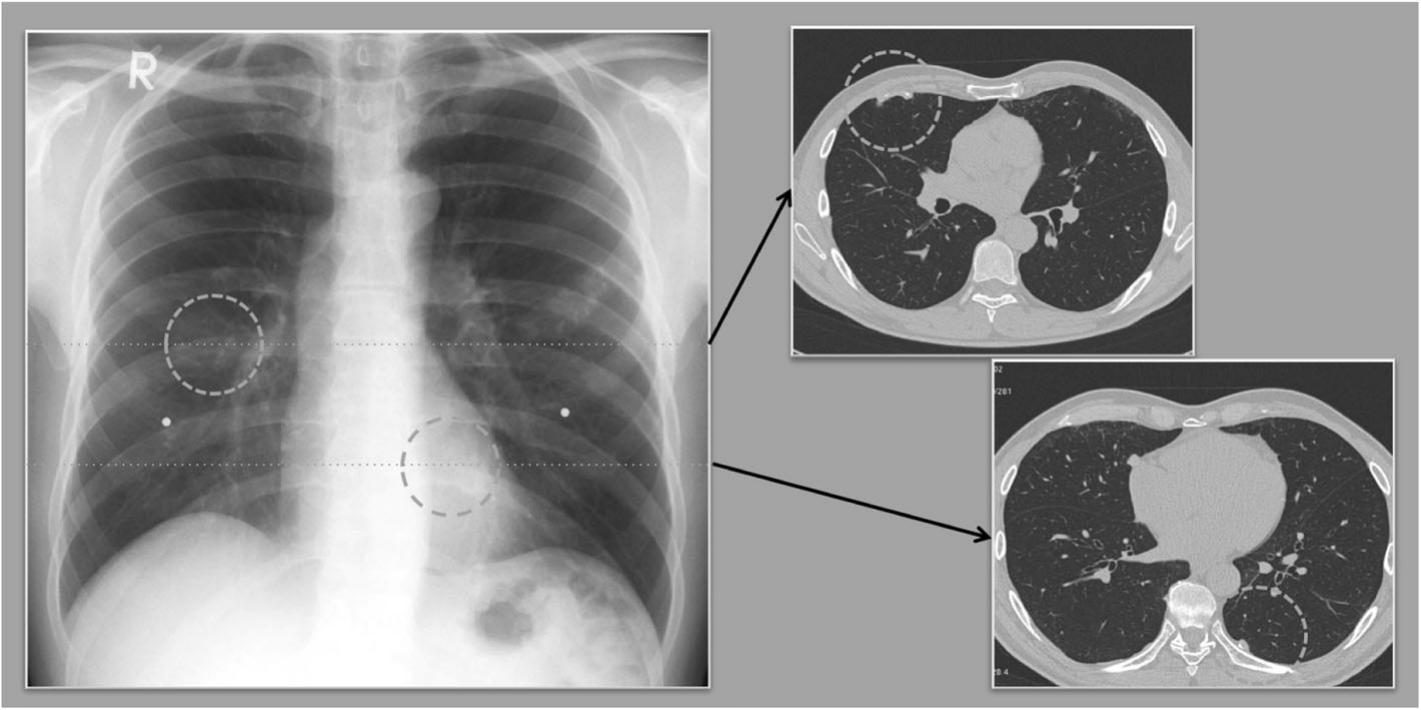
Comparison analog/digital Chest Radiograph versus Computed Tomography, findings on the pleura are overlooked or in face-on projection confused with tumor (Source: Prof. Dr. Kurt Georg Hering)
